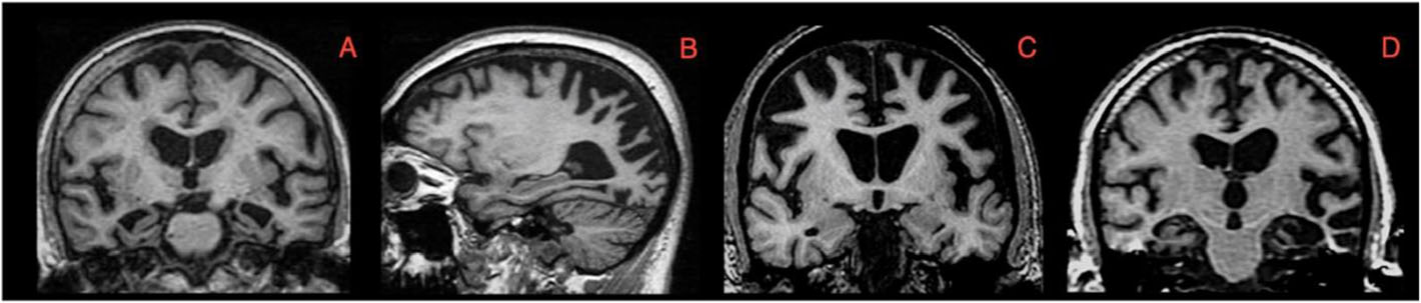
T1-weighted MRI scans demonstrating characteristic cortical atrophy signature in selected diseases: a typical amnestic Alzheimer’s disease, b posterior cortical atrophy, c behavioural variant frontotemporal dementia and d semantic dementia
